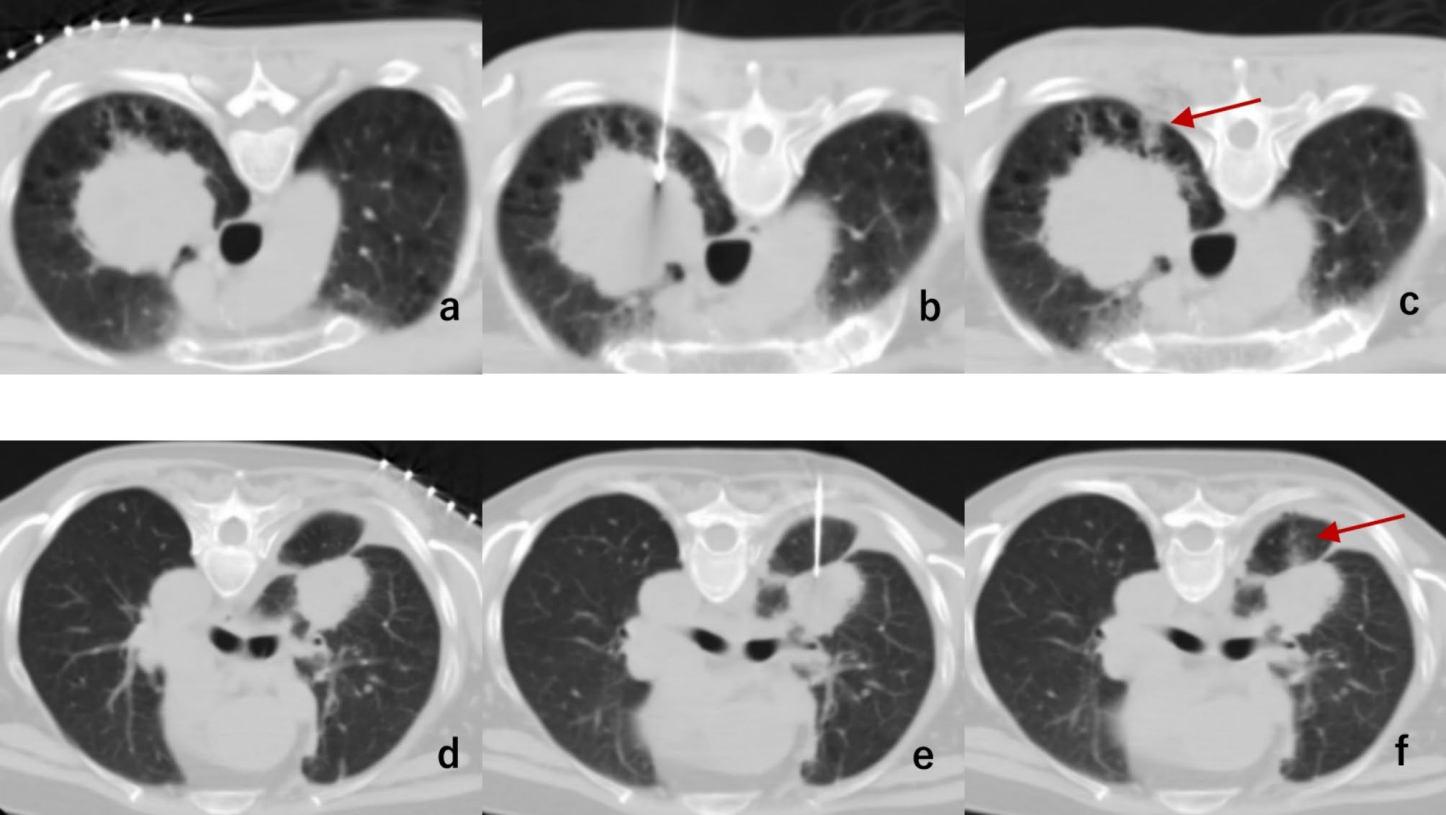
Axial computer tomography (CT) fluoroscopic image of 2 lung biopsy procedures with tract sealing. A 59 (Y/M) patient with emphysema and a suspicious left upper lobe lung lesion (a-c), and another 67(Y/F) patient with a right upper lobe lung lesion contact with fissure (d-f). Image showing the pulmonary bullae surrounding the lesion (a), the trocar needle incidentally transgressed the bullae (b). withdraws the needle to a 5–10 mm subpleural area and injects the sponge particle, forming a well-defined opacity(arrow). No pneumothorax was detected after the needle was removed (c). Image showing the lesion contact with fissure (d), the trocar needle passed the fissure (e), tract sealing was performed, and no pneumothorax was detected after the needle was removed (f)
